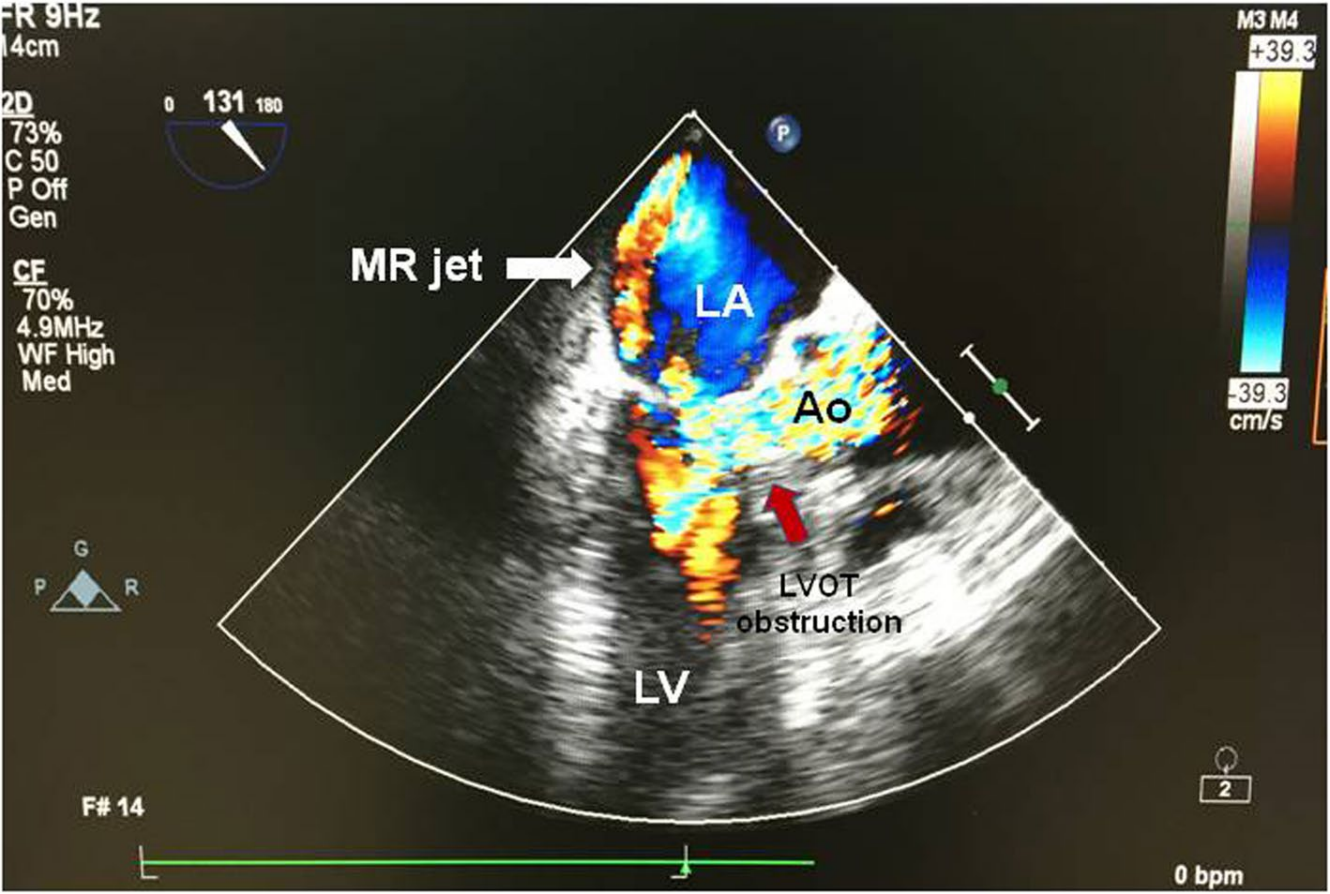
Intraoperative transesophageal echocardiography with color-flow Doppler mapping (midesophageal long axis view) showed a significant MR jet (white arrow) and mosaic flow signals in the left ventricular outflow tract (red arrow). LA = left atrium; LV = left ventricle; LVOT = left ventricular outflow tract; MR = mitral regurgitation; Ao = aorta. An additional movie file shows this in more detail (see Additional file 1)
